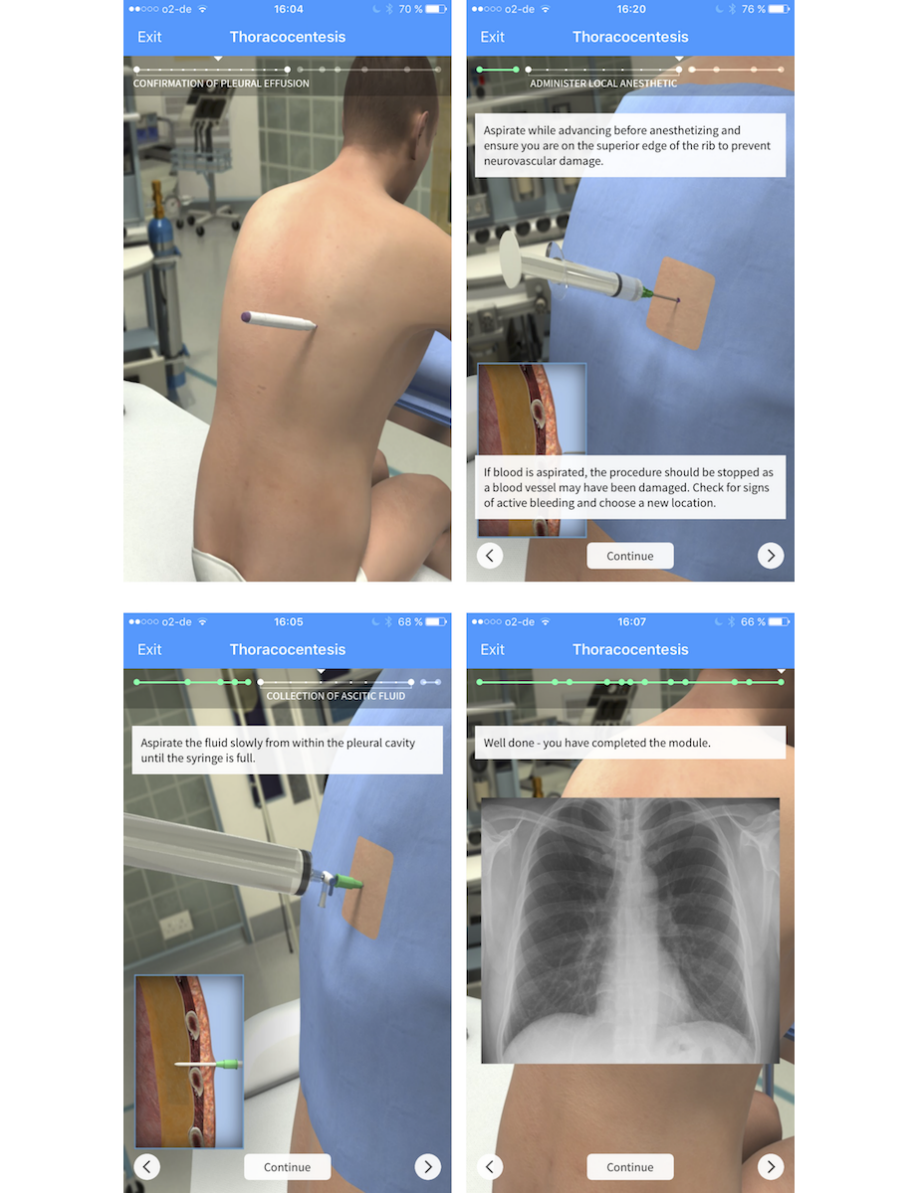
Screenshots of the Touch Surgery “Thoracocentesis” module. Panels A to D visualize the different key steps of the module. A: anatomical location of approach; B: correct administration of local anesthetics; C: aspiration of fluid; D: radiological control using a chest X-ray.
